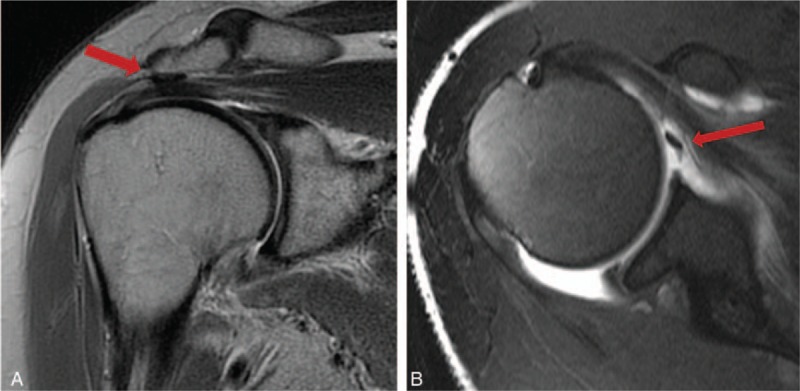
A, Preoperative T2-wighted MR image of soft tissue super in subacromial space (arrow). B, Preoperative MR arthrogram image of ruptured cord like MGHL. MGHL = glenohumeral ligament, MR = magnetic resonance.
